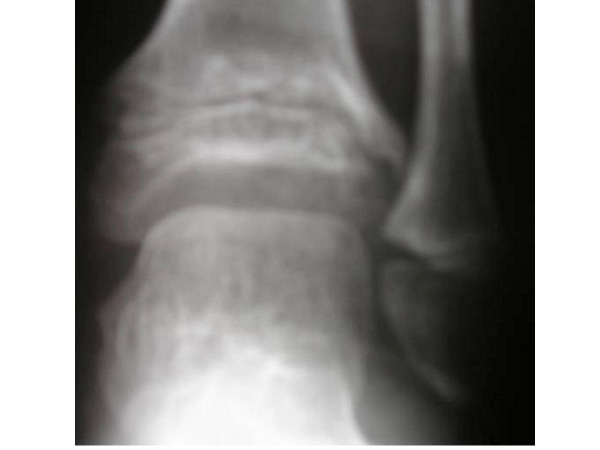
Anteroposterior radiograph of the ankle joint showing enchondromas with the appearance of linear lucencies. The chondrocytes appear to line up in a vertical orientation along the epimetaphyseal components associated with sclerosis of the articular surface.
